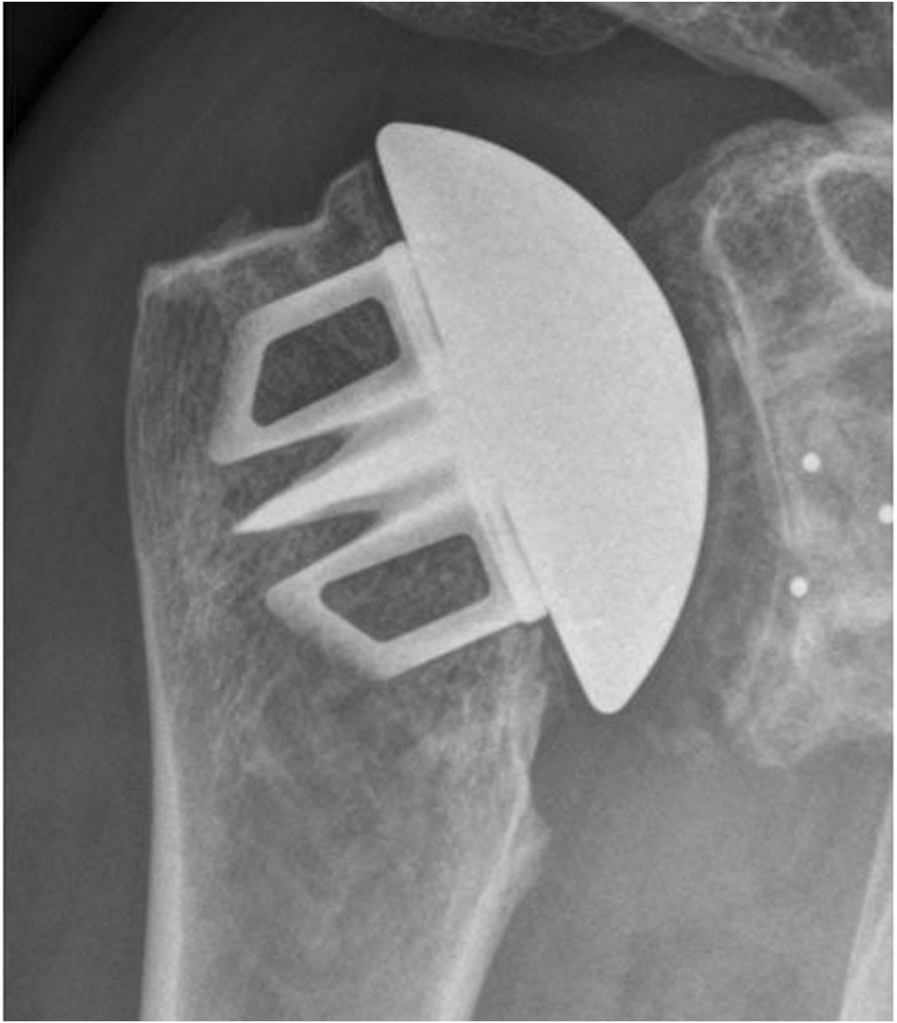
Radiograph shoulder in true-AP: Sidus with calcar resorption
